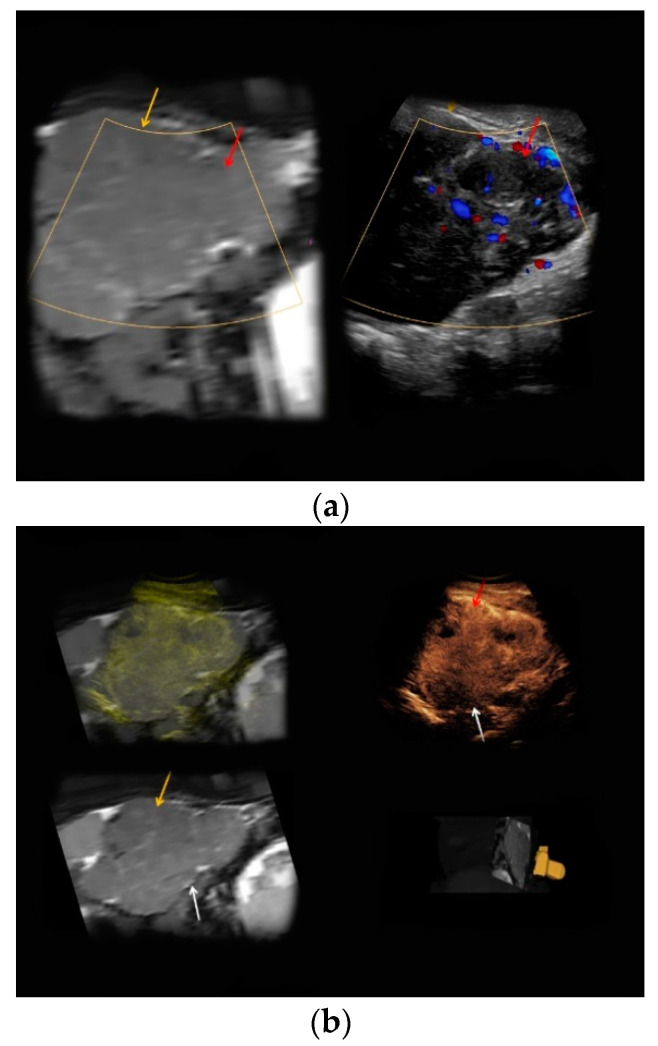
Renal angiomyolipoma, 25 weeks of pregnancy, real-time MRI-/CEUS-Fusion Imaging. (a) T2-hypointense tumor (yellow arrow) with 14 cm in diameter of the left kidney in unenhanced MRI (left, red arrow—renal parenchyma), T2-weighed image, axial reformation; displayed in a side-by-side manner with corresponding Doppler mode, which revealed no lesional hypervascularization (right, yellow arrow) compared to adjacent renal parenchyma (right, red arrow). (b) Wash-out during the late phase of the tumor was detected during CEUS (upper right, white arrow), no wash-out of the renal parenchyma (red arrow); correlate of unenhanced MRI (lower left, yellow arrow—renal parenchyma, white arrow—renal tumor); fused correlates of CEUS and MRI (upper left); real-time 3D navigation of the CEUS-/MRI-Image Fusion (lower right).
